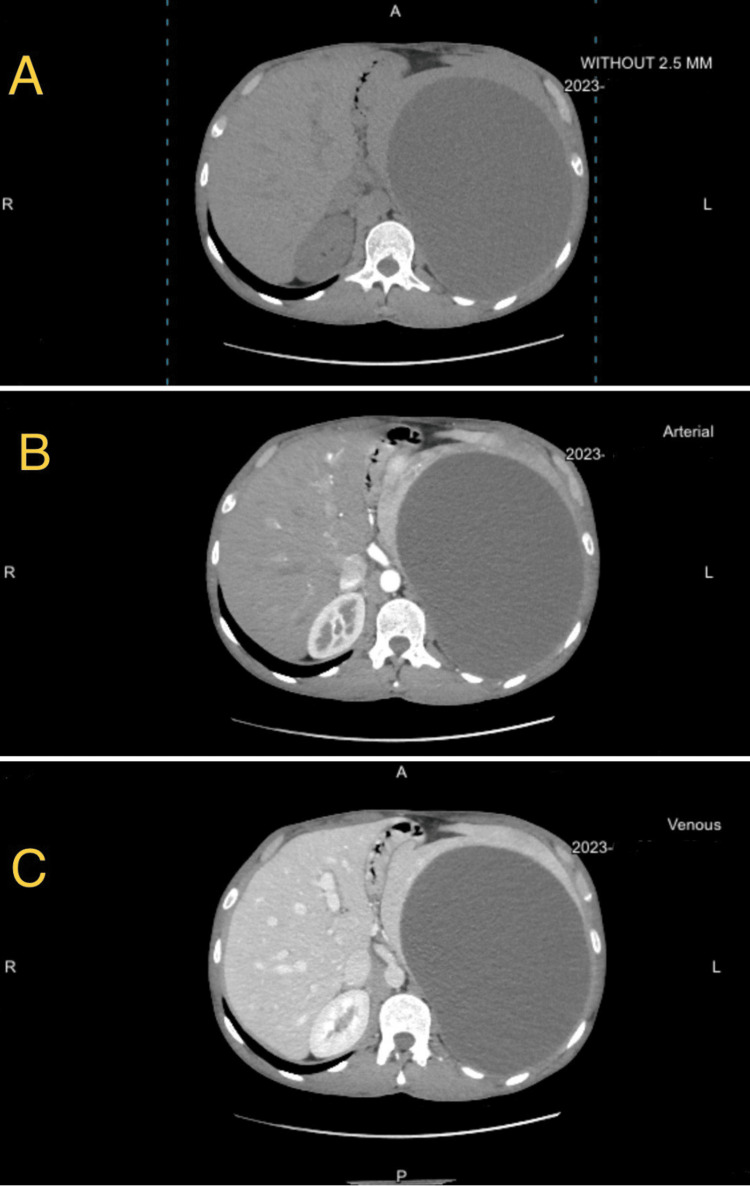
Abdominal CT scan. A. Pre-contrast. B. Arterial phase. C. Venous phase. CT, computed tomography
